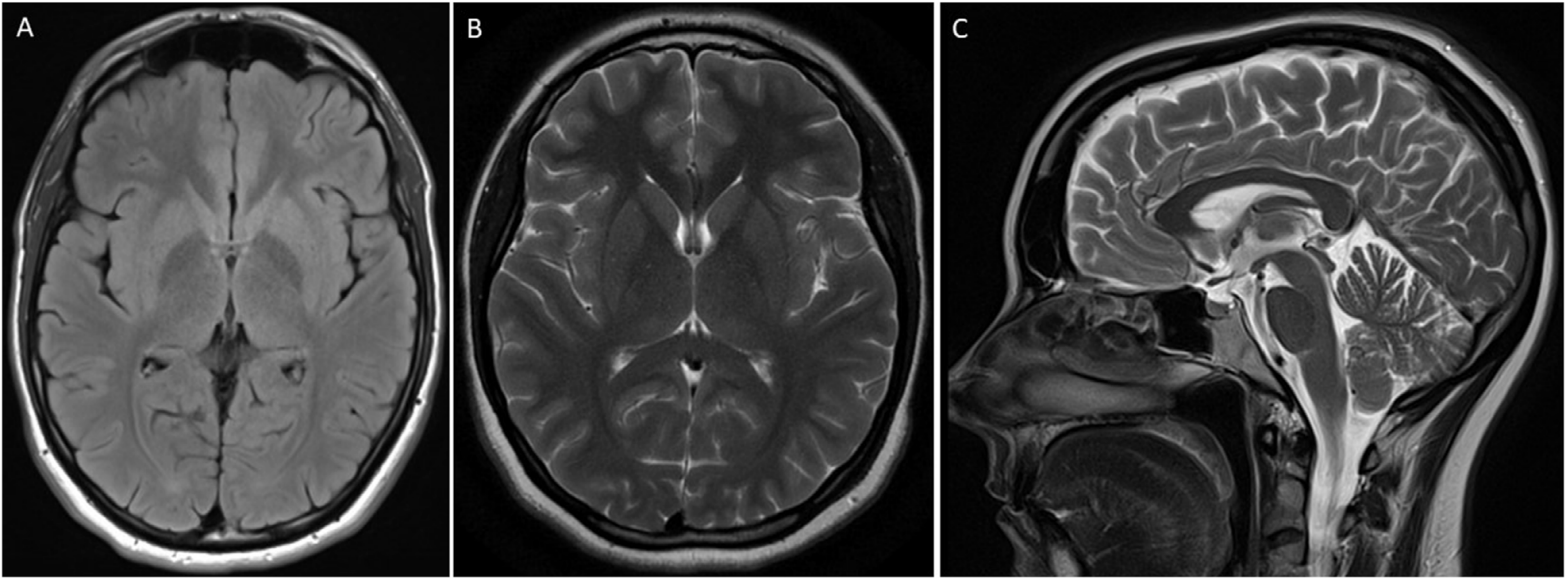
MRI of the brain of an individual showing features of Parkinson’s disease. T2 flair axial (A), brain T2 (B), and brain Sagittal T2 (C) were unremarkable.
